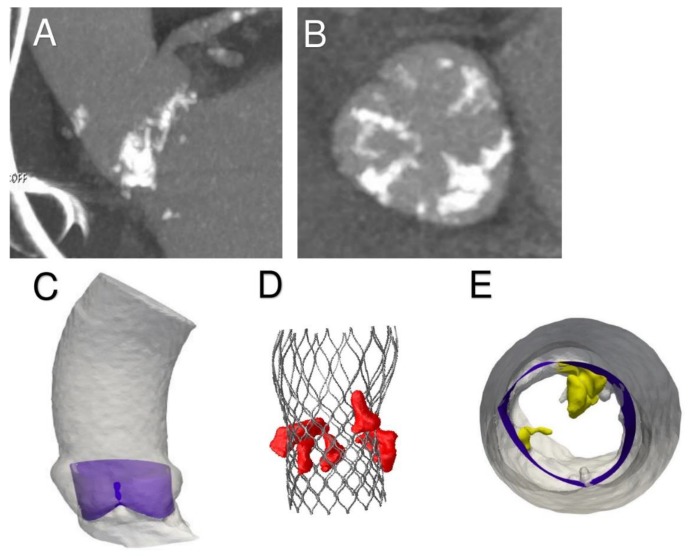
(A,B) Preoperative CT scan shows massive calcifications of aortic leaflet and root. (C) Extraction of aortic root geometry. (D,E) 3D preoperative model: aortic wall with leaflets and calcific blocks.
